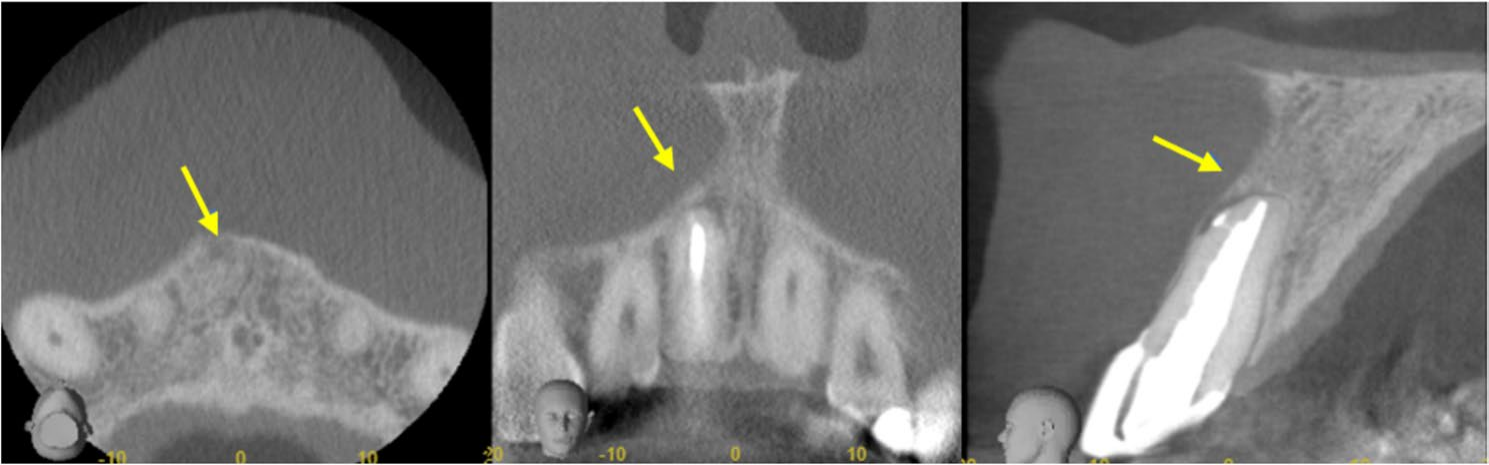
Three-axis CBCT view of the periapical region at 6-month follow-up. The yellow arrow indicates the previously affected area, now showing signs of bone regeneration and periapical healing following endodontic treatment and surgical intervention
